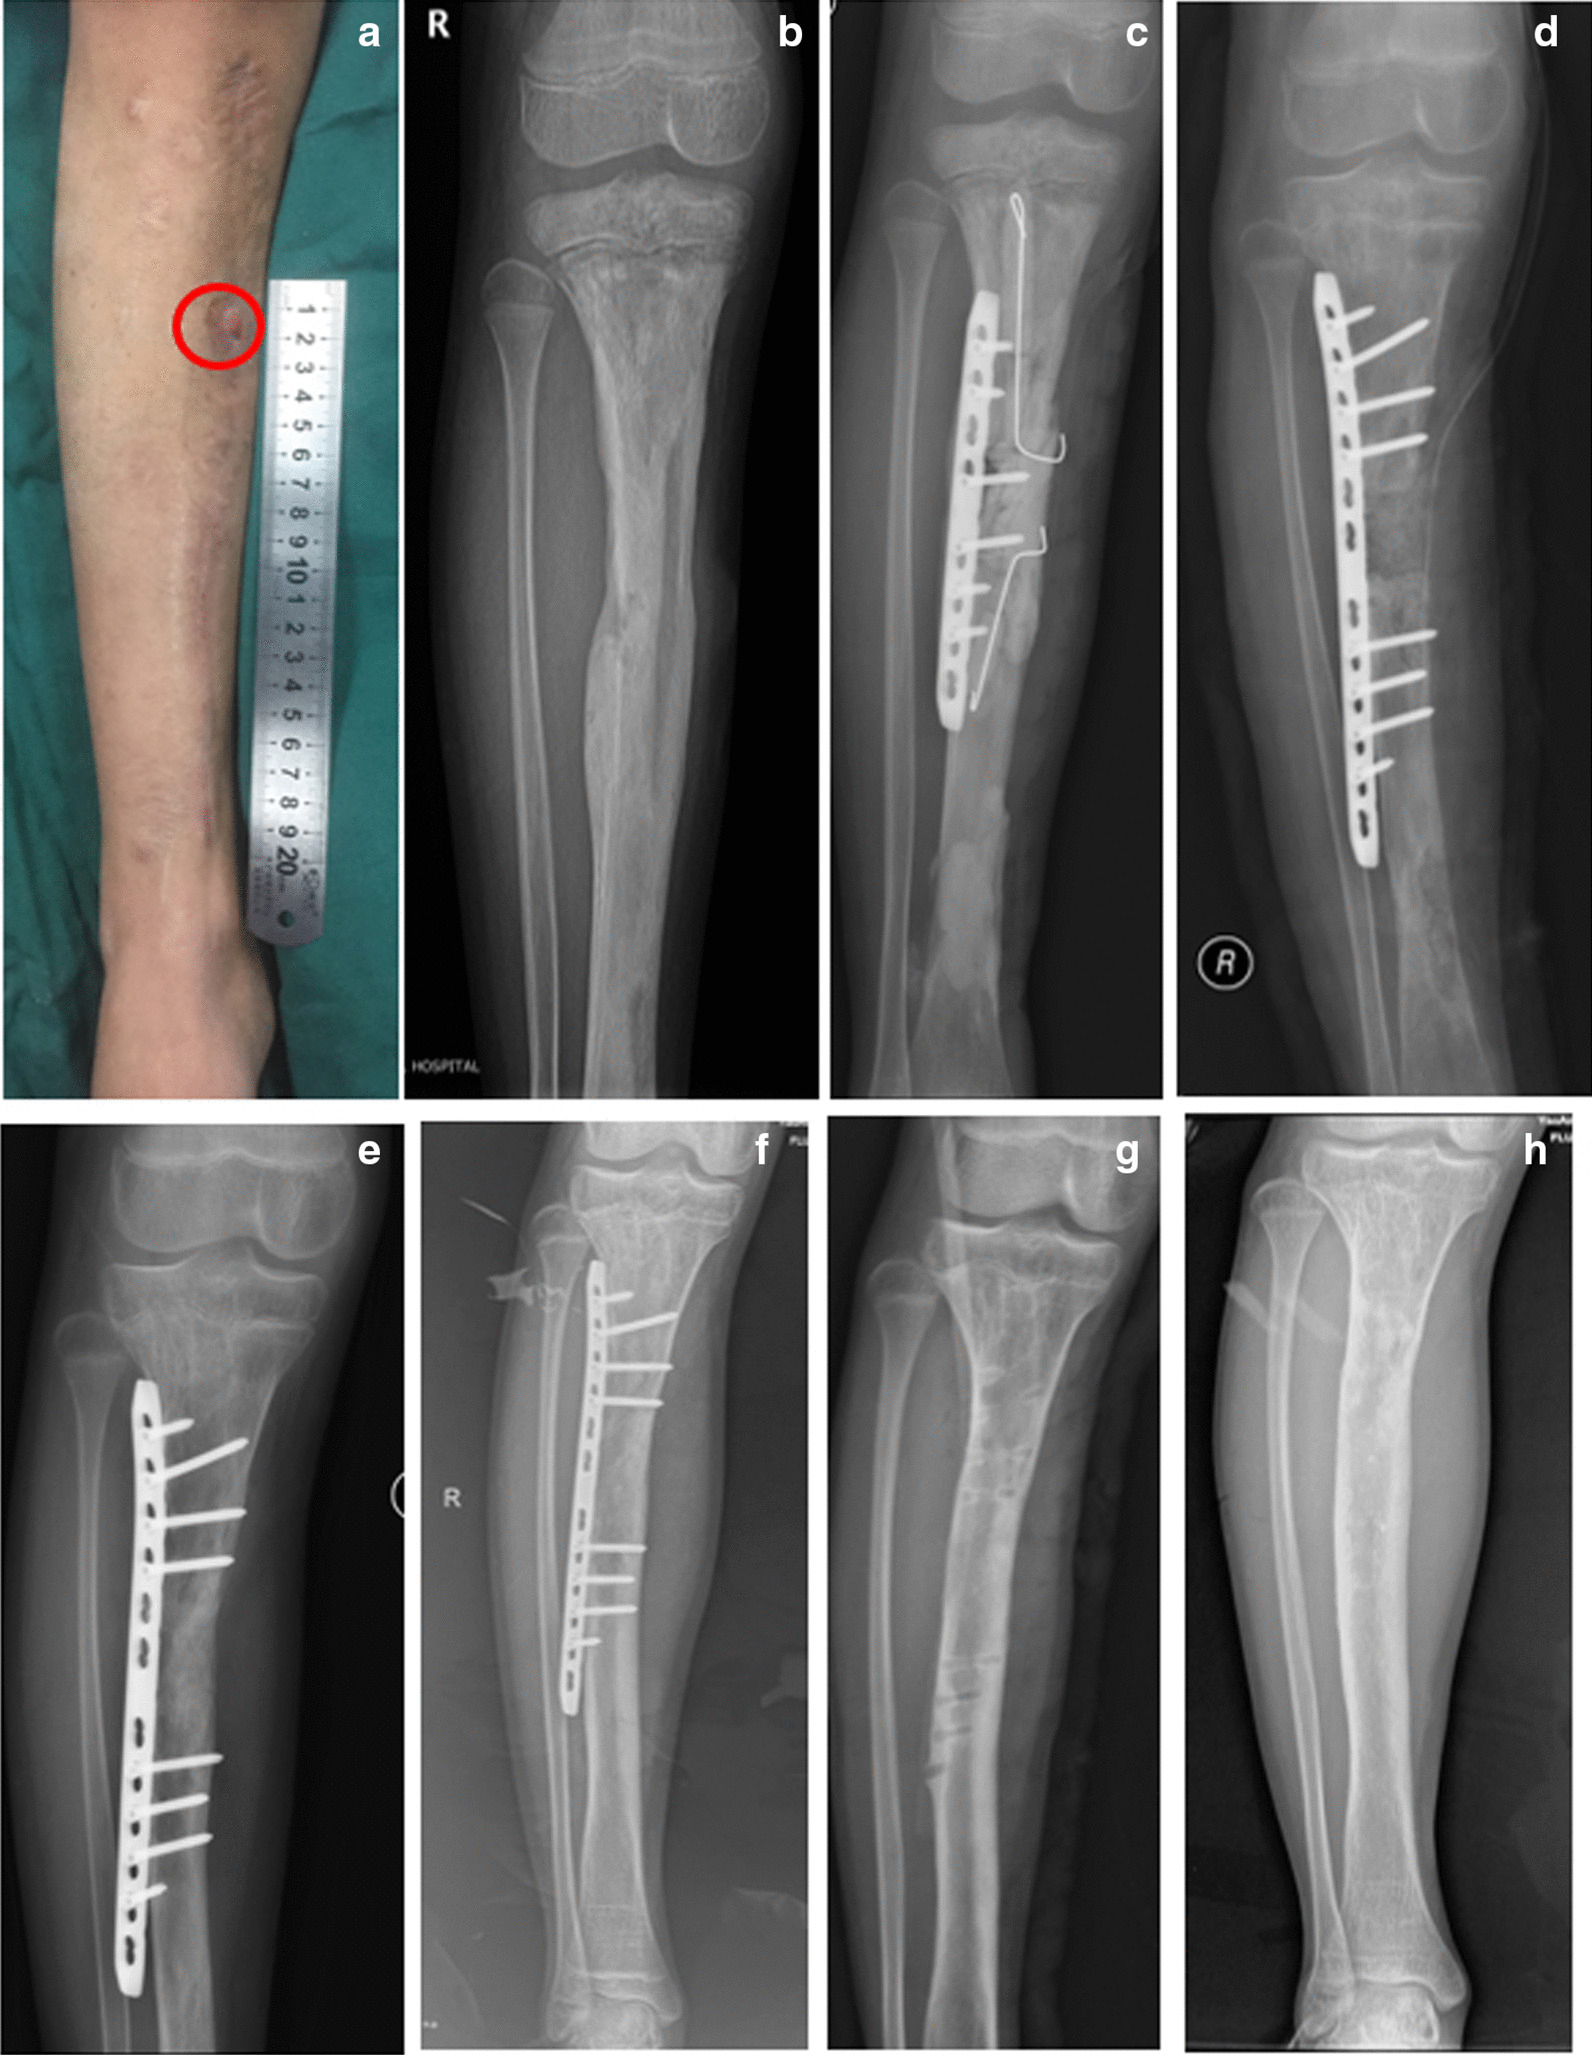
Case 19: a 10-year-old boy with recurrent pain and drainage from his right tibia. Three months before, he underwent surgery twice due to swelling, redness, and pain with no preceding trauma. However, the drainage from sinus tract (red circle) and pain did not disappear after the operations. a Pre-operative presentation of the right lower leg. b Anteroposterior (AP) radiograph shows chronic osteomyelitis of the right tibia. c In the first-stage surgery, debridement and internal fixation were performed, and antibiotic-loaded bone cement was used to fill the bone defect and medullary cavity. d AP radiograph after bone graft in the second stage of surgery. e AP radiograph shows bone defect healed at 3 months after bone grafting. f AP radiograph shows bone defect remodelled at 23 months after bone grafting. g AP radiograph shows that internal fixation was removed at 26 months after bone grafting. h AP radiograph shows the right tibia at 47 months after bone grafting
